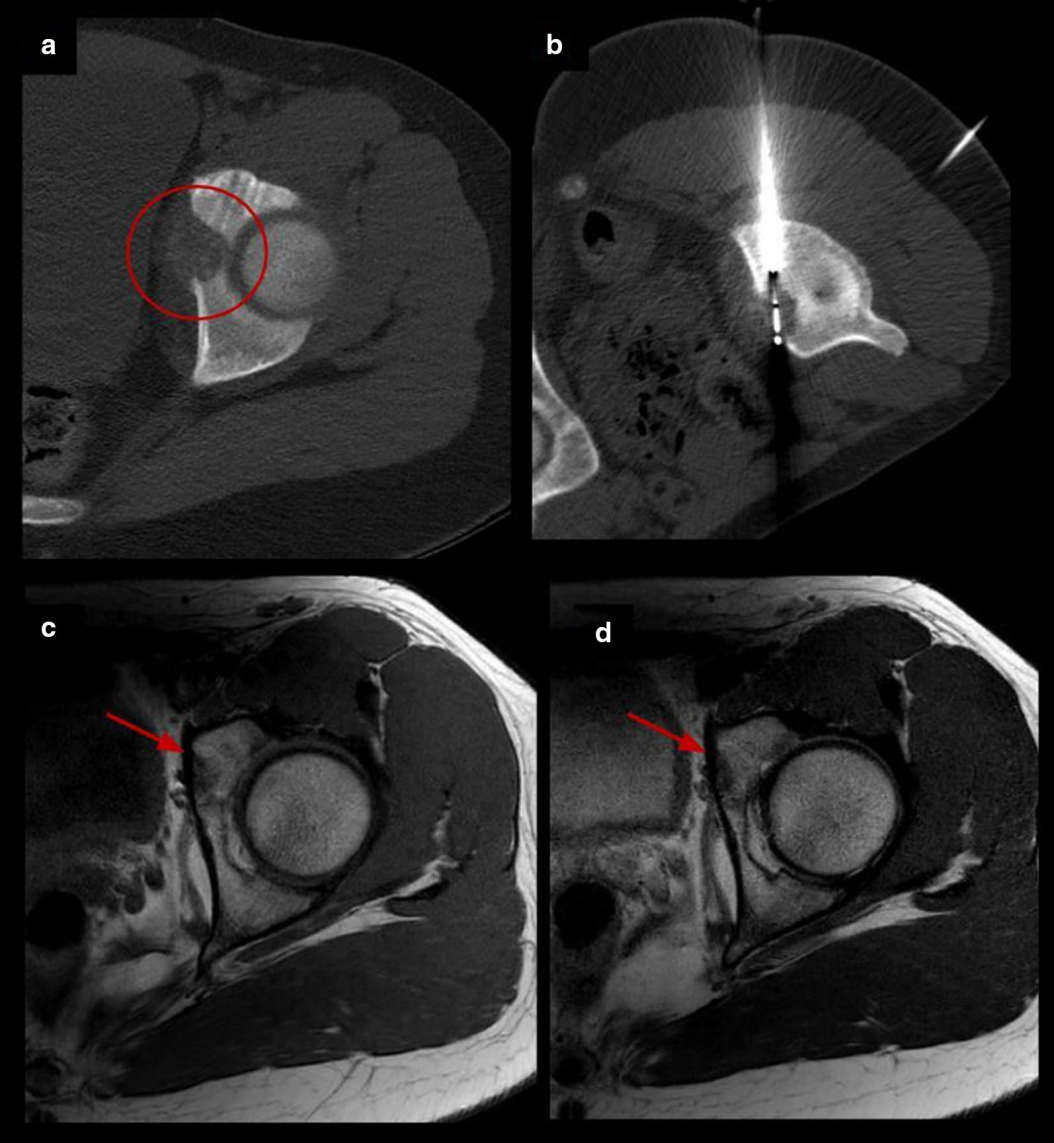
Acetabulum osteoblastoma cryoablation. a Axial CT images demonstrating an expansive osteolytic tumor with partial matrix mineralization in the left acetabulum corresponding to osteoblastoma (circle). b Axial CT exhibiting one cryoprobe placed within the nidus. c, d Follow-up MRI images 15 months after treatment with complete resolution of the lesion (arrows)
